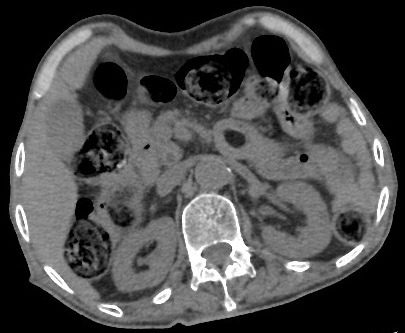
Abdominal non enhanced helical CT. Transverse axial scan showing an oval hypodense lesion typical of fatty tissue, surrounded by the wall of proximal jejunum
